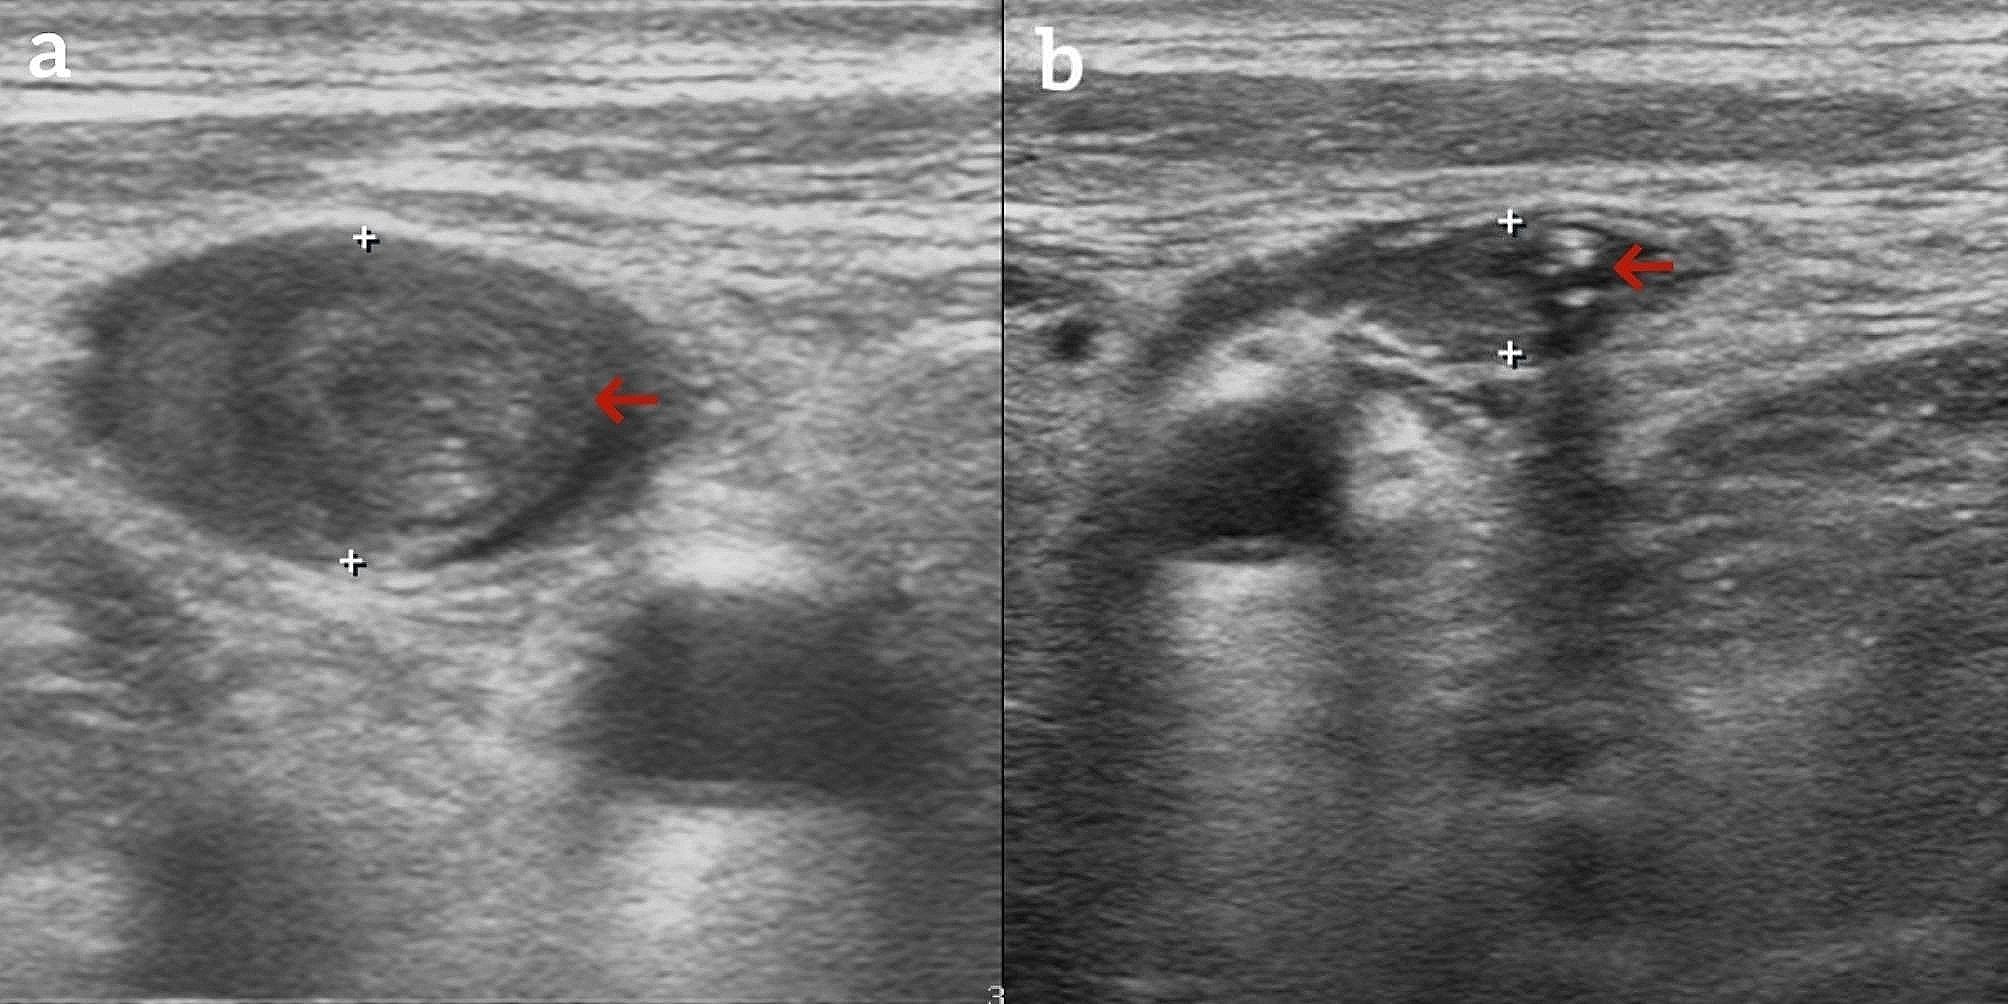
Internal jugular vein ultrasound shows thrombosis in bilateral internal jugular vein. a: thrombus (red arrow) in right internal jugular vein; b: thrombus (red arrow) in left internal jugular vein
